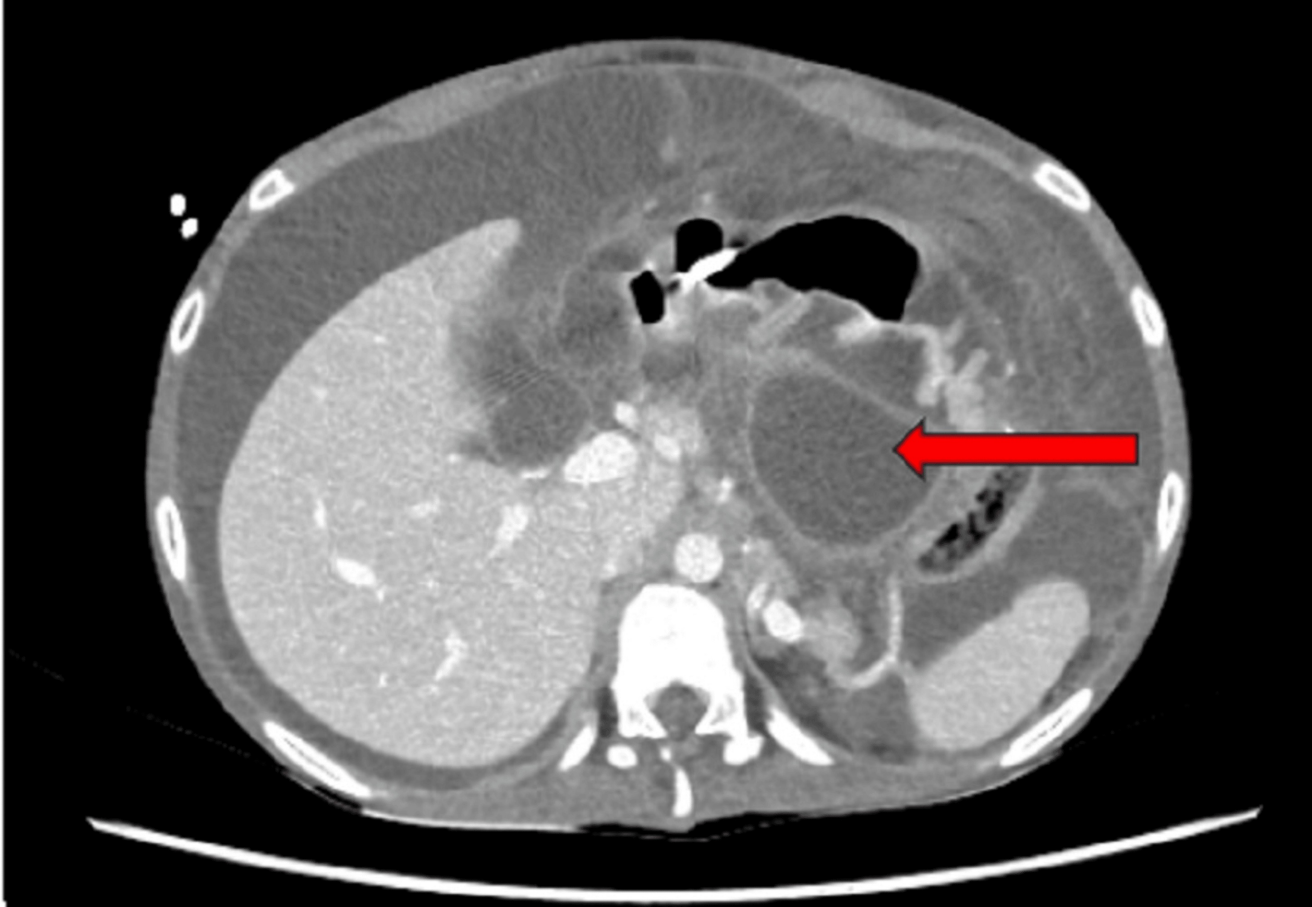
Abdominal CT scan showing pseudo-pancreatic cyst (arrow) CT: computed tomography
